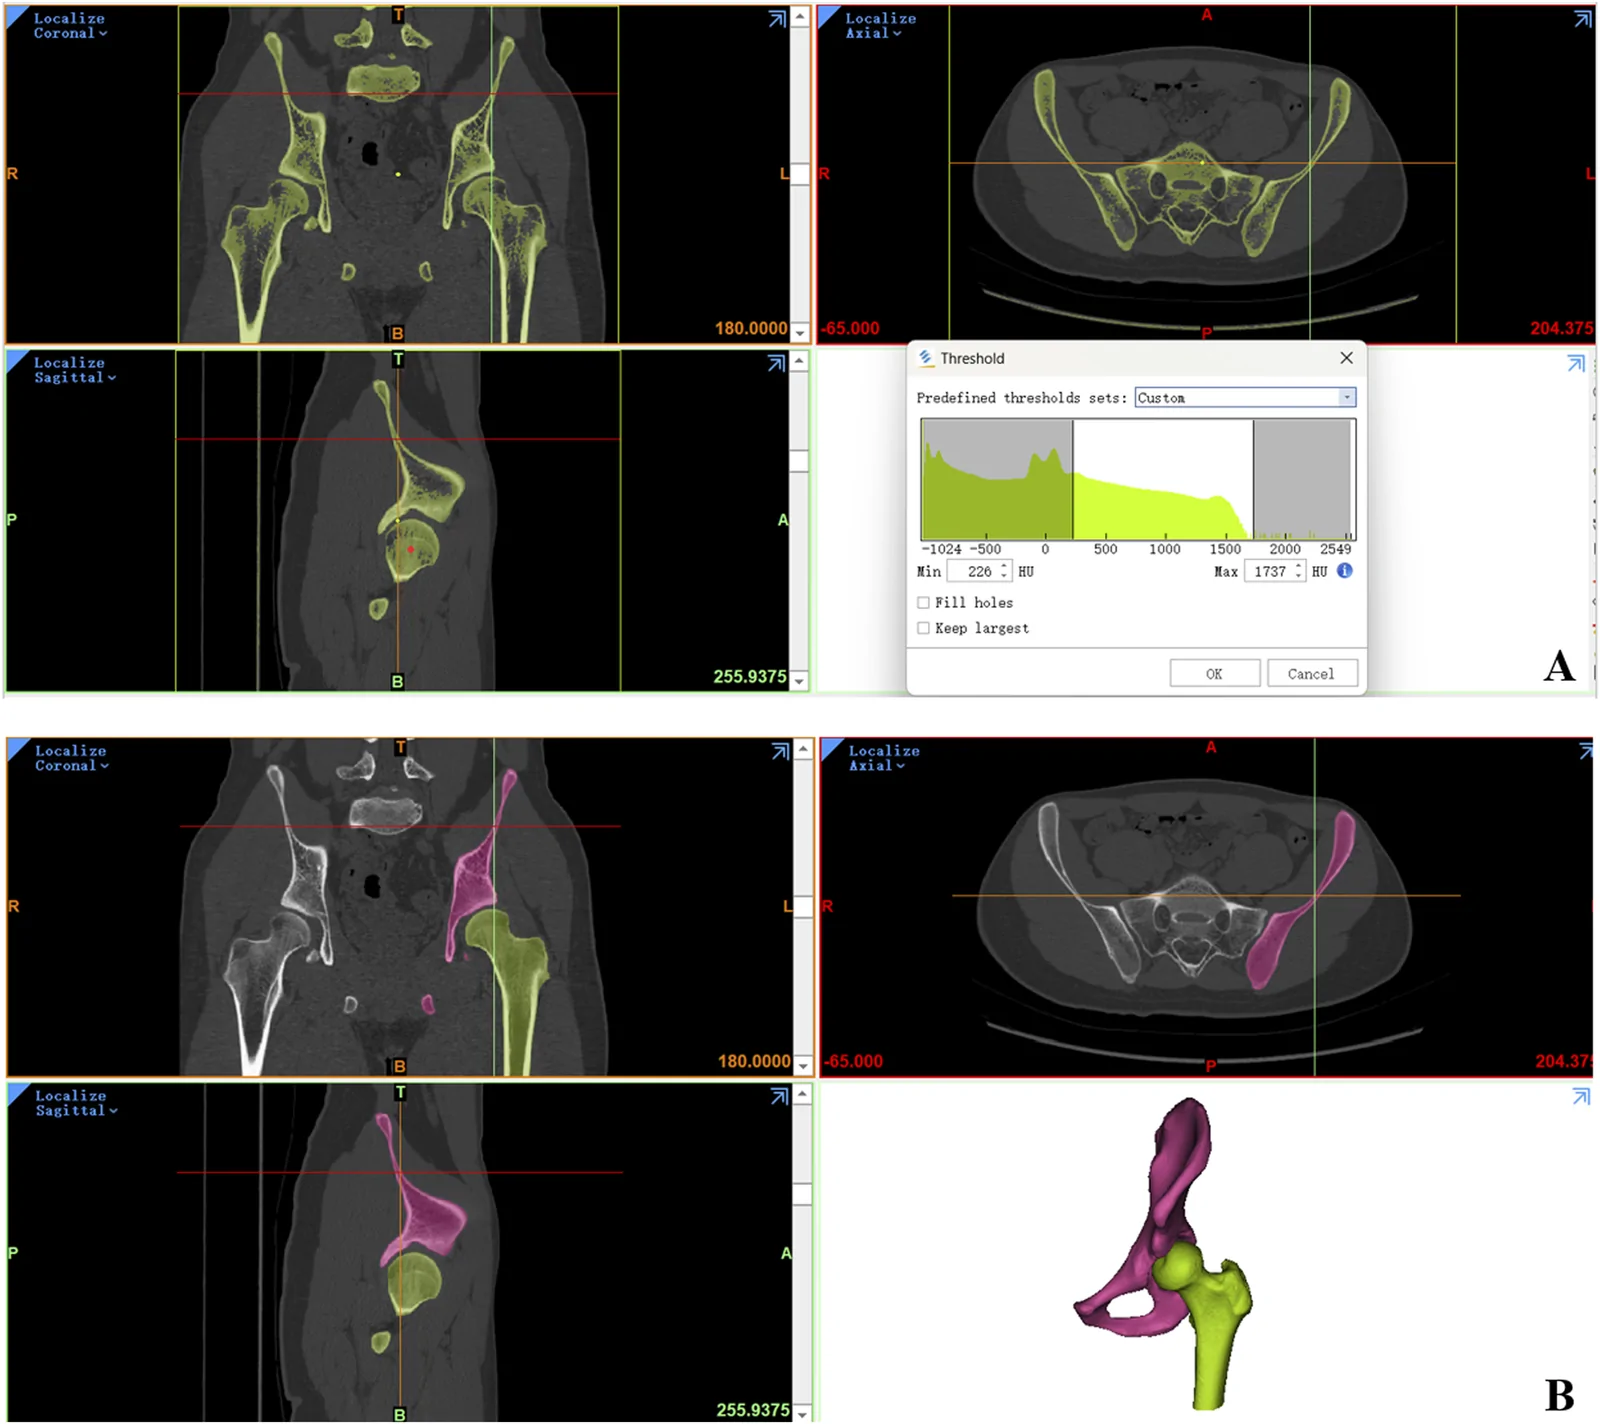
Original image segmentation and bony three-dimensional reconstruction. (A) Import of the original CT images. (B) Segmentation of the pelvis and femur with initial three-dimensional reconstruction.
